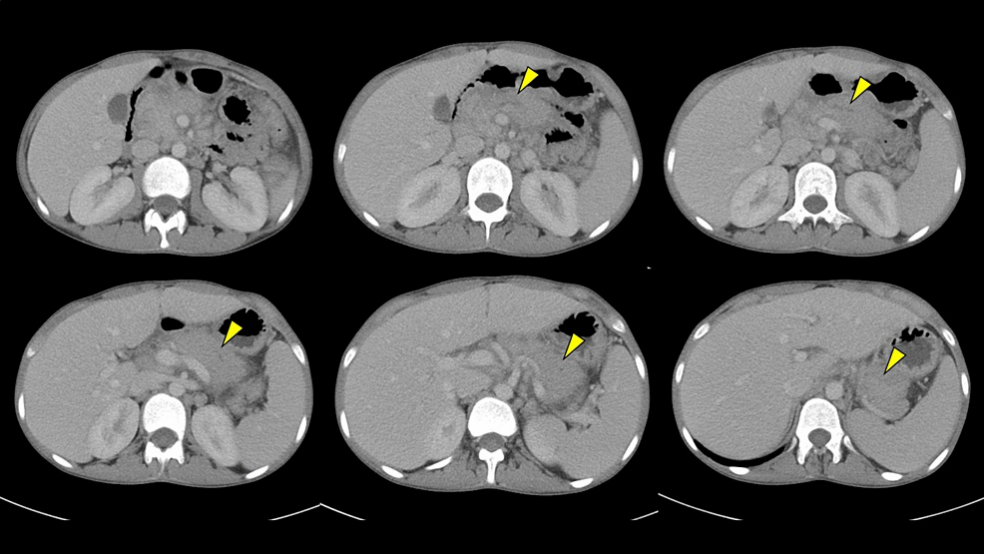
Contrast-enhanced computed tomography scan Contrast-enhanced computed tomography (CT) showed swelling of the pancreas with inflammation spreading to surrounding areas and dilation of the main pancreatic duct in the pancreatic body-tail (yellow arrow).
